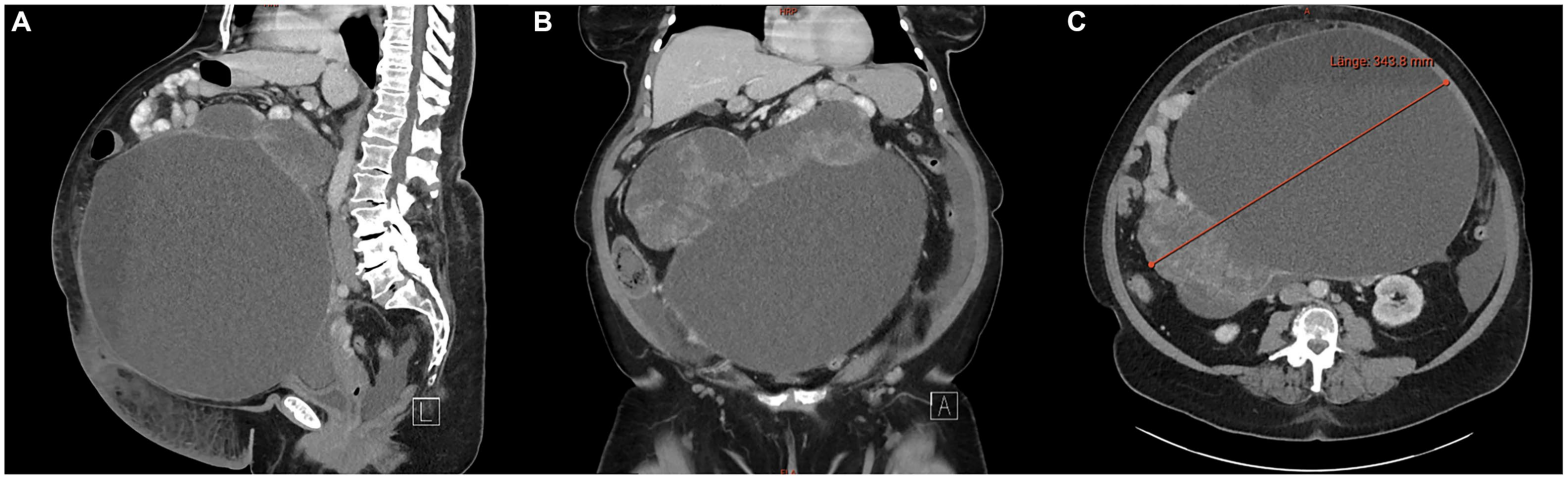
Contrast-enhanced computed tomography (CT) of the abdomen and pelvis reveals a giant, predominantly cystic right ovarian mass measuring approximately 35 cm in diameter, with multiple septations and fat-fluid levels, suggestive of a teratomatous lesion. (A) Sagittal view; (B) coronal view; (C) axial view.
